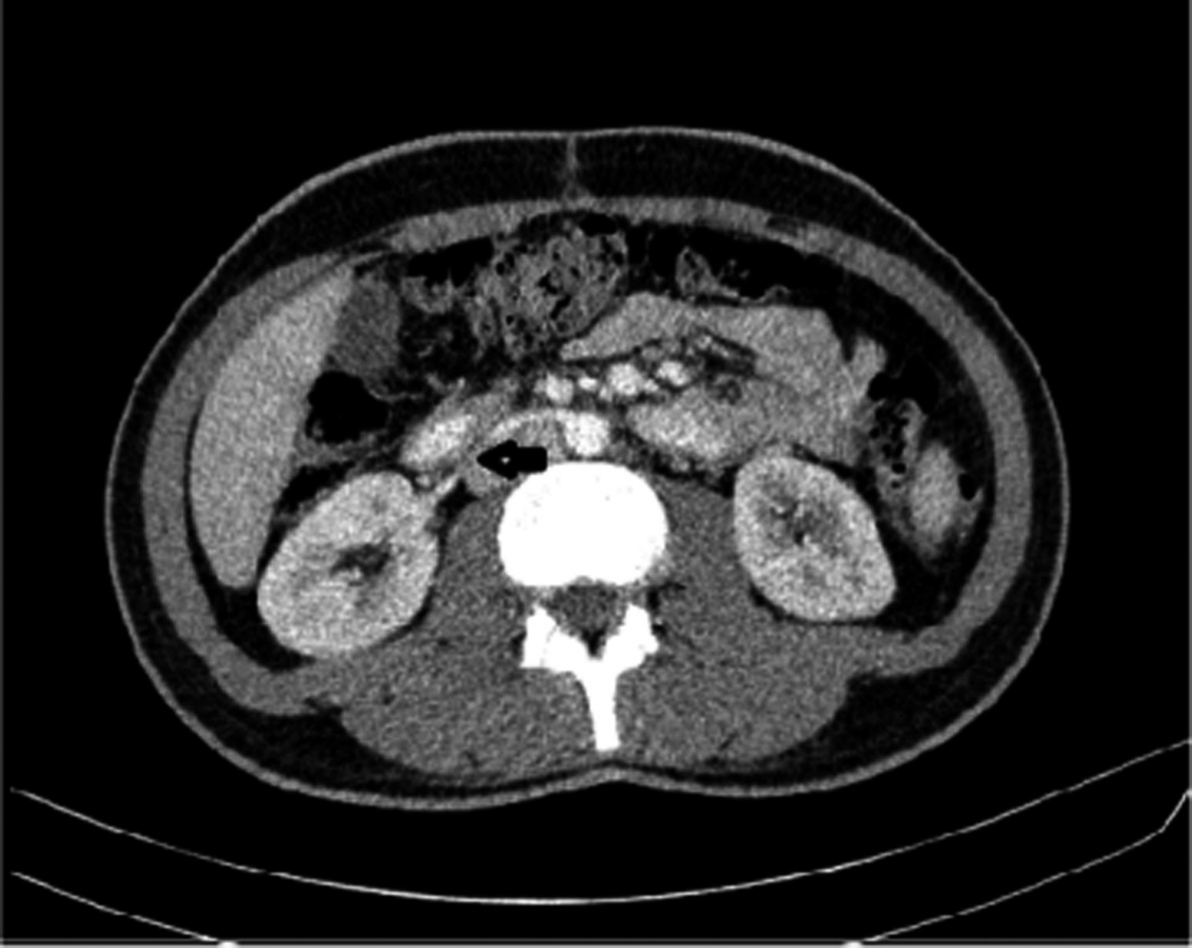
Contrast enhanced Computed tomography scan depicting solitary right renal artery. P, Pre caval right renal artery
